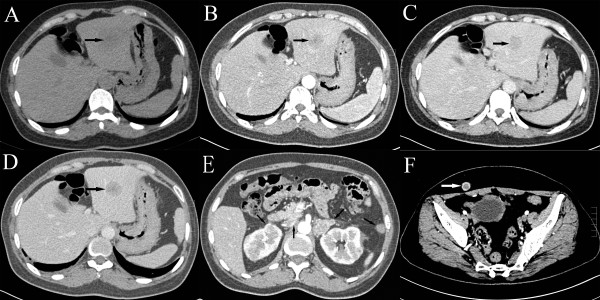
Abdominal CT. A-D) Plain scanning and triphasic contrast-enhanced scanning of lesions in the left liver lobe. E) Adrenal gland and kidney lesions. F) Lesions in the subcutaneous fat layer.
